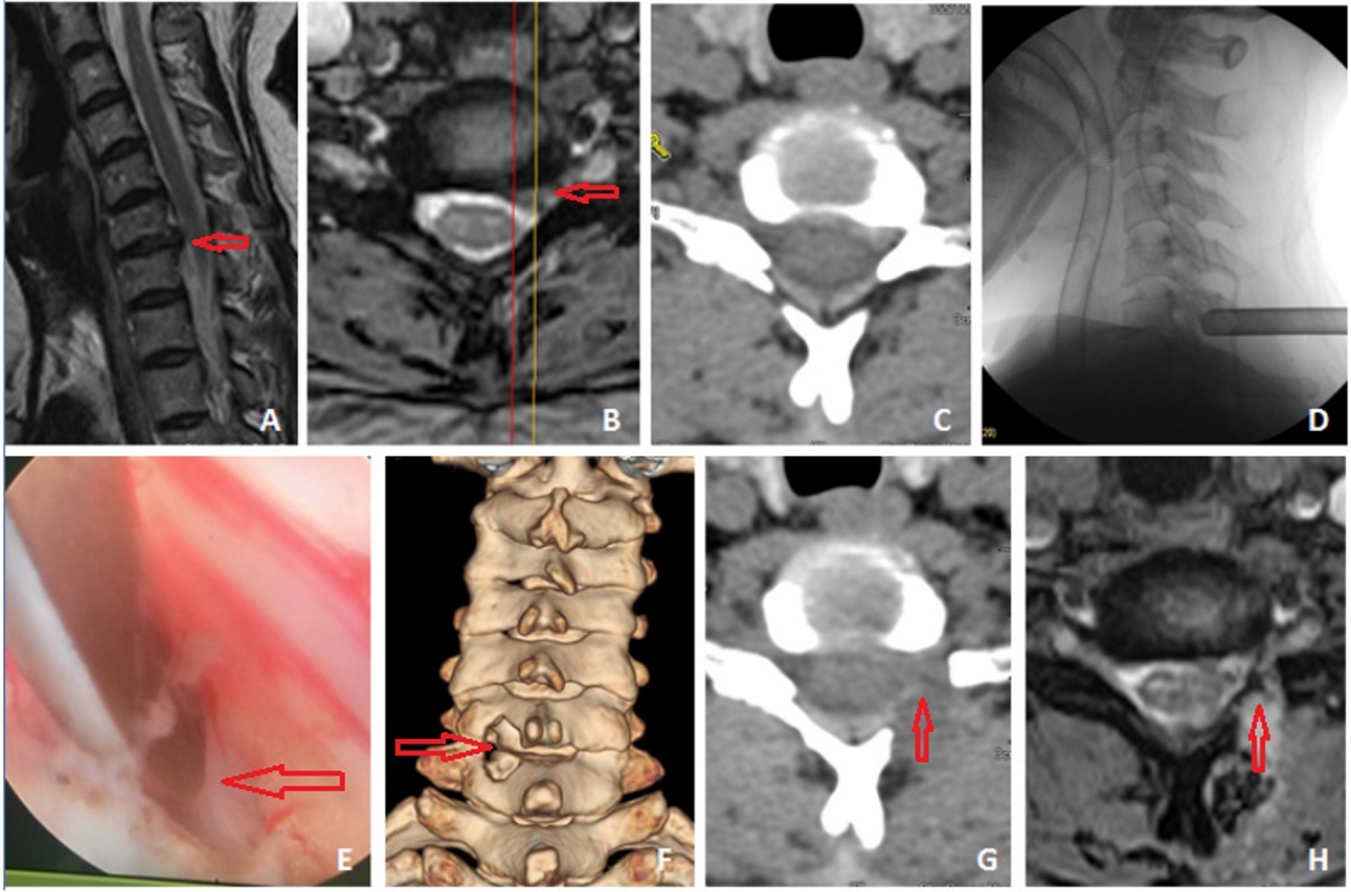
A patient with CSR (C6/7 left foraminal bony stenosis), female, 52 years old, was treated with posterior cervical full-endoscopic foraminotomy and decompression. Preoperative MRI and CT showed that C6/7 intervertebral disc protruded to the left rear, combined with left foraminal bony stenosis (A–C). After intraoperative localization, excising the herniated nucleus pulposus, cutting off the posterior osteophyte, performing foraminotomy and decompressing the nerve roots (D,E). Postoperative three-dimensional reconstruction CT showed a keyhole like decompression window in the left lamina of C6/7 (F) The postoperative axial CT and MRI showed satisfying decompression. The osteophytes and herniation had been removed, and the intervertebral foramen had been fully expanded (G,H).
